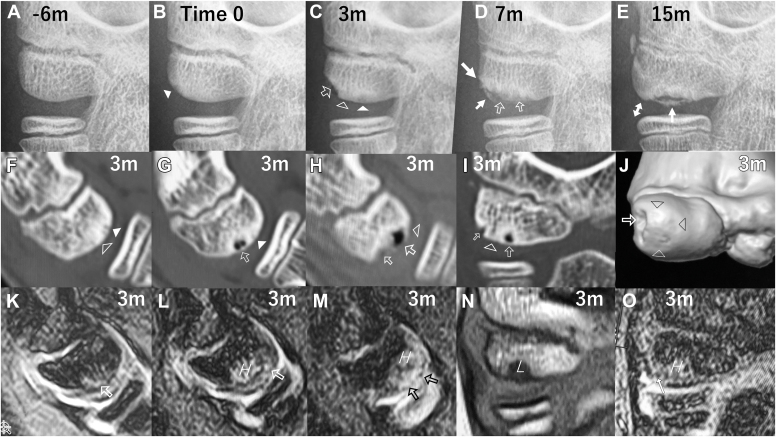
Case 2, a 11.7-year-old boy with osteochondritis dissecans of the capitellum. (A-E) Anteroposterior radiographs of the elbow at 45° of flexion (APR45). (A) APR45 at initial presentation showing no abnormal findings of the capitellum. (B) APR45, taken 6 months after initial presentation, showing the first detectable feature that was extremely subtle rarefaction surrounded by sclerosis with a limited area of the ossifying subchondral bone surface in the lateral aspect of the capitellum (*arrowhead*). This time was assumed to be Time 0. (C) APR45, taken 3 months after Time 0, showing subchondral bone rarefaction (*arrowhead*), flattening (*open arrowhead*), and depression (*open arrow*) surrounded by osteosclerosis. He was diagnosed to have OCD and treated nonoperatively. (D) APR45, taken 7 months after Time 0, showing enlargement of subchondral bone depression (*open arrows*) surrounded by uneven osteosclerosis in the central aspect of the capitellum and 2-way ossification from the underlying bone (*large white arrow*) and along the original capitellar bone surface (*small white arrow*). (E) APR45, taken 15 months after Time 0, showing union in the lateral aspect of the capitellum (*double arrow*) and nonunion in the central aspect (*arrow*). (F-O) CT images and MRI taken 3 months after Time 0. (F, G, H, I) Sagittal CT images, reconstructed along a plane with 10° in valgus,^41^ and coronal CT image, reconstructed along a plane with a tilting angle of 55°,^41^ showing flattening (F, H, I: *open arrowhead*) and subchondral bone cysts (G, H, I: *open arrows*) surrounded by osteosclerosis. (J) 3D-CT image showing a partial bone defect (*open arrow*) and flattening (*arrowheads*). (K, L, M) Sagittal MRI taken along a plane with 10° in valgus.^41^ (N, O) Coronal MRI taken along a plane with a tilting angle of 55°.^41^ (K, L, M, O) Fat-suppressed T2-and T2∗-weighted MRI showing focal high signal lesions (K, L, M, *open arrows*), a high-signal interface (O, *white arrow*), and high signal area (*H*) consistent with bone effusion. (N) T1-weighted MRI showing low signal area (*L*) consistent with osteogenic changes and bone defect. *CT*, computed tomography; *MRI*, magnetic resonance imaging.
